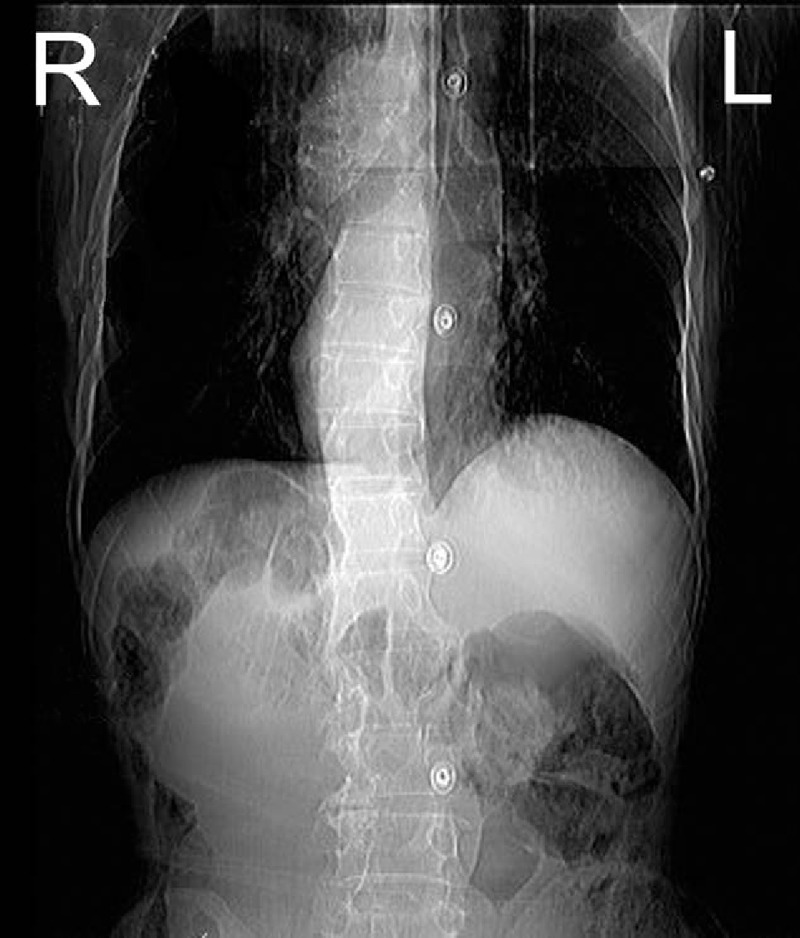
Plain film showed a left–right transposition of all viscera and dilated intestine.
